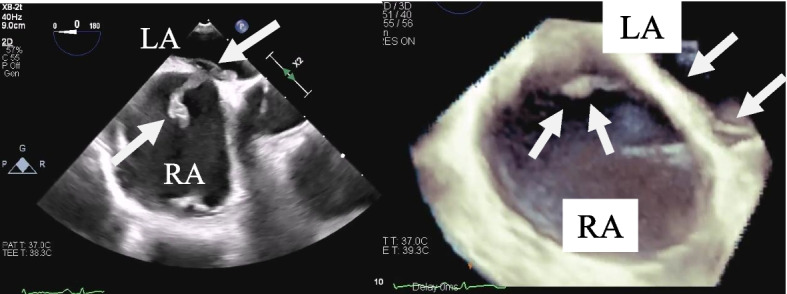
Transesophageal echocardiography showing a thrombus protruding into the left atrium through the patent foramen ovale and fluttering in both atria (arrow: thrombus, RA: right atrium, LA: left atrium)
